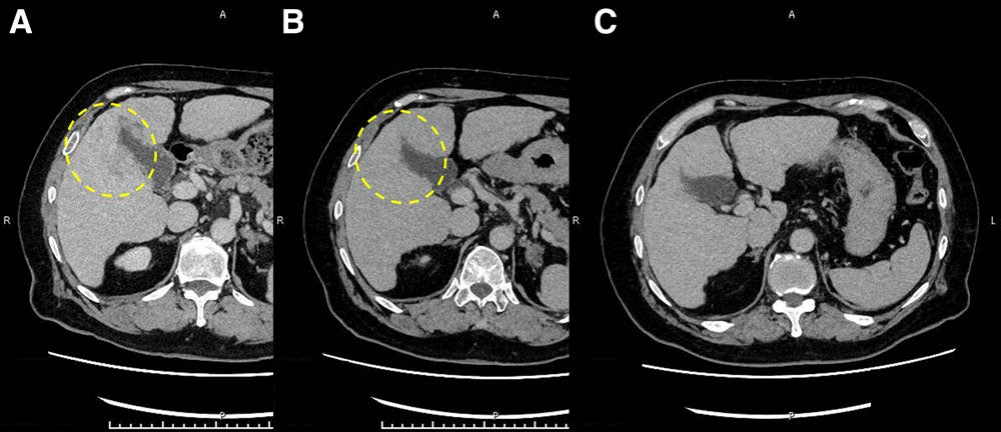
Imaging changes of the main tumor in computed tomography (equilibrium phase). (A) Image at the first diagnosis (32 days after the first visit); (B) the tumor shrank and became obscure (139 d after the first visit); (C) the tumor disappeared completely (334 d after the first visit).
